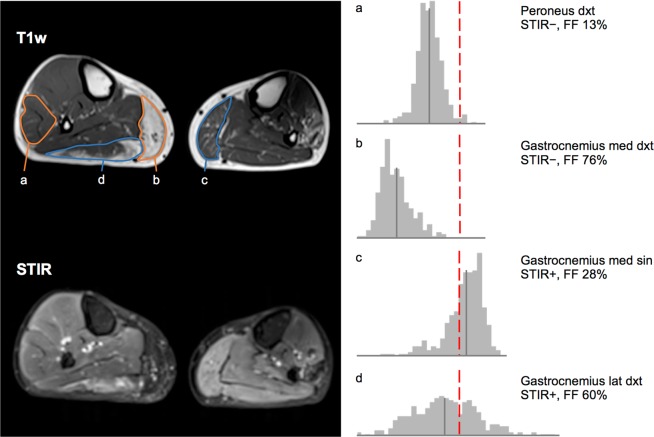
STIR sequences of muscles. T1-weighted (T1w) and STIR images of calf muscles in a patient with FSHD. Pixel by pixel histograms of the distribution of STIR signal intensities of four muscles are shown to the right. Their mean signal intensity is marked by a filled gray line in each histogram. The right peroneus muscle (a) is STIR− and has normal fat fraction. The histogram of all signal intensities is normally distributed. The right gastrocnemius medialis (b) is also STIR− but is severely fat replaced. The histogram is negatively skewed and the mean signal intensity is correspondingly lower. The left gastrocnemius medialis (c) is STIR+ and has normal fat fraction. Compared to muscle (a), the histogram is positively skewed resulting in an increased mean signal intensity that corresponds to the higher water fraction. The right gastrocnemius lateralis (d) is also STIR+ but is severely fat replaced. The combination of high water and fat content results in mean signal intensity similar to muscle (a). The percentage of pixels above the upper reference limit (red dashed line) better corresponds with the water content.
